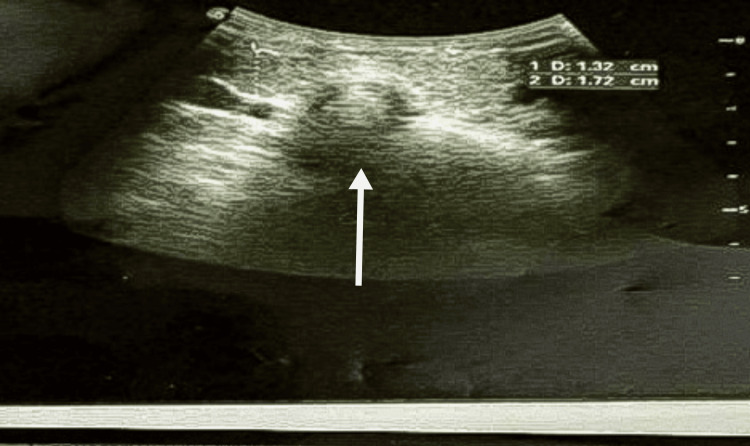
Ultrasonography of the suprasternal swelling.
